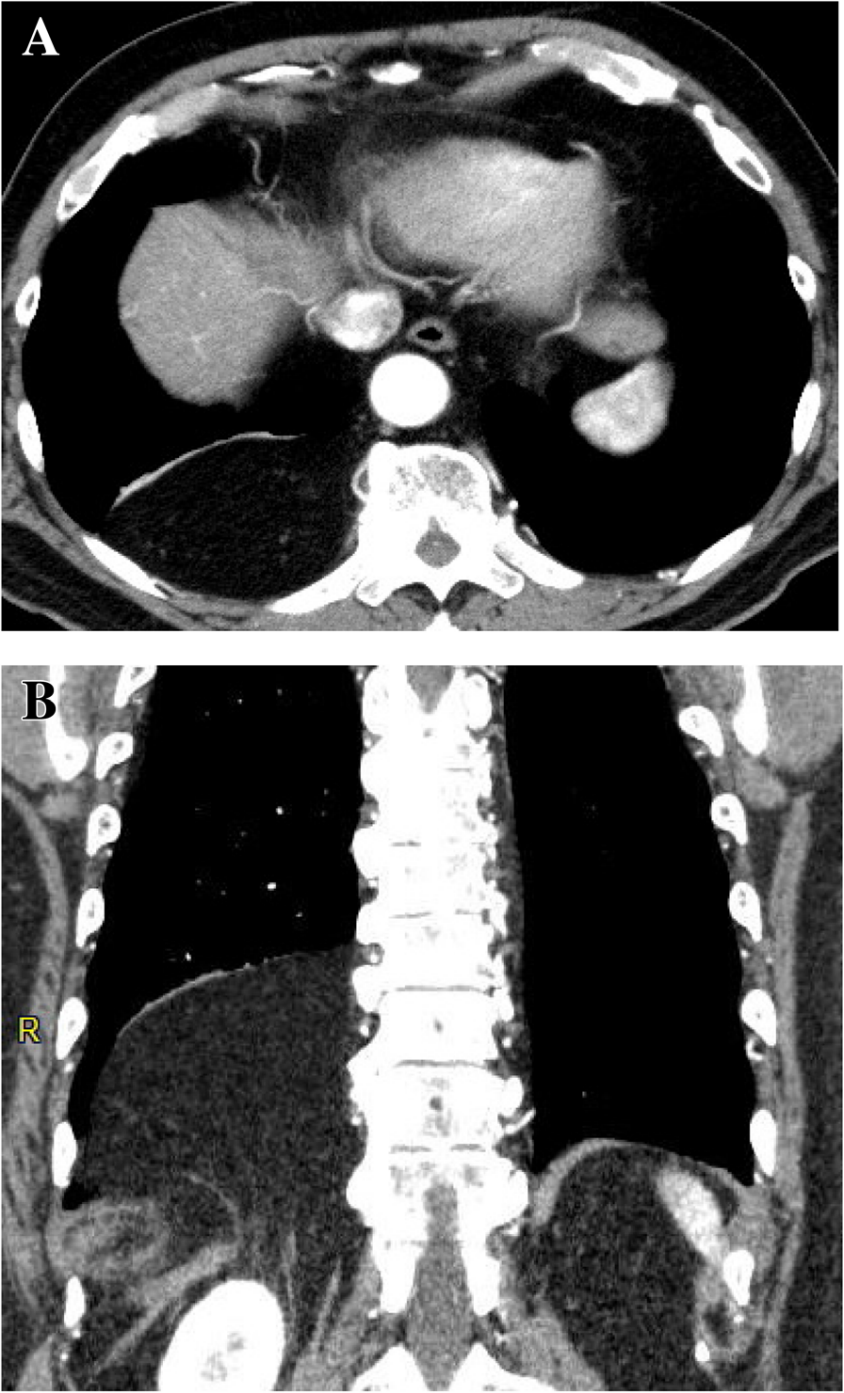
Chest CT images showing a a well-circumscribed mass in the right dorsal thoracic space measuring 28 × 9× 10 cm and compressing the right lower lobe. b The tumor is mostly composed of fatty density and seemed to connect with retroperitoneal fat
